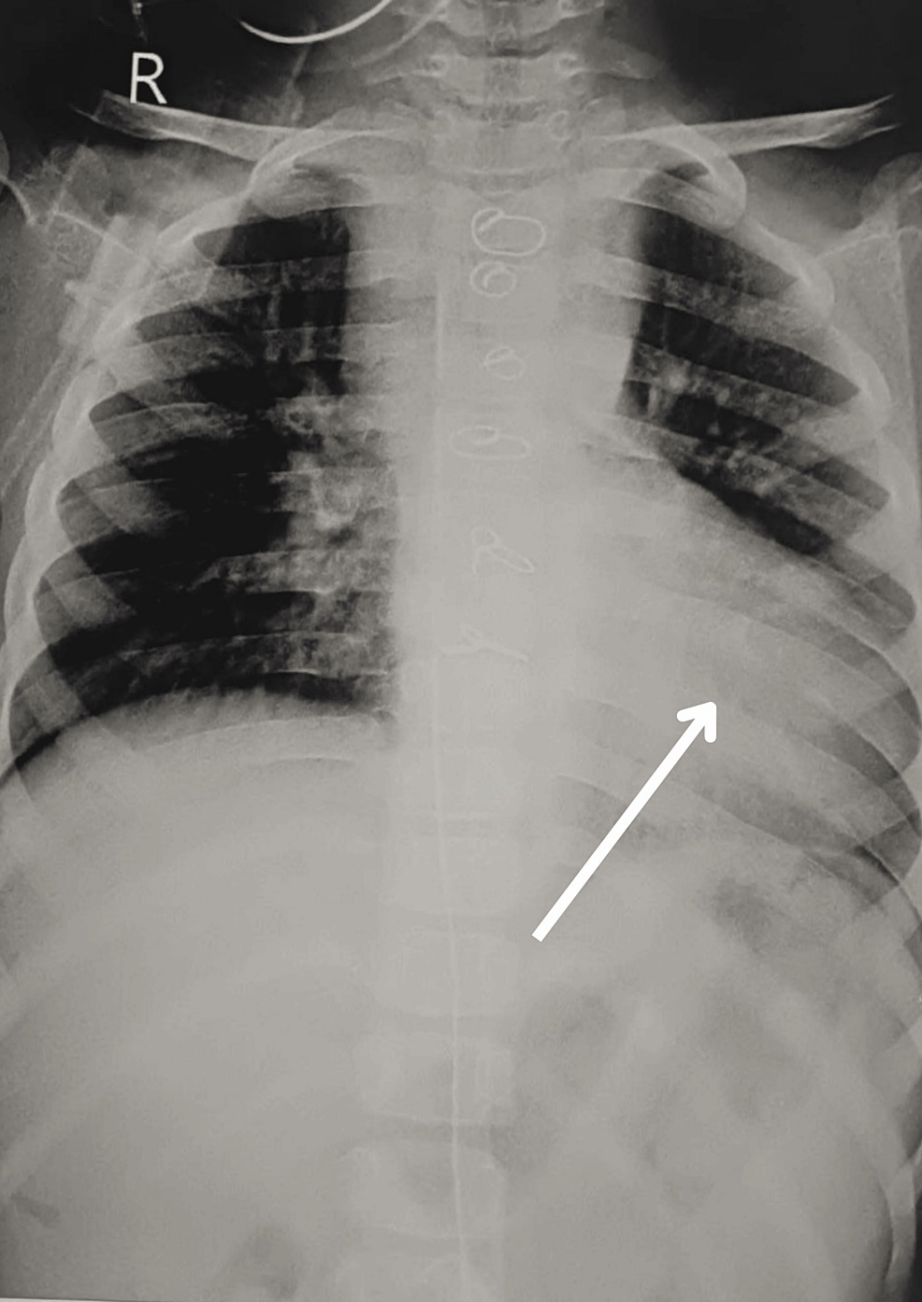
Chest X-ray The white arrow indicates cardiomegaly
